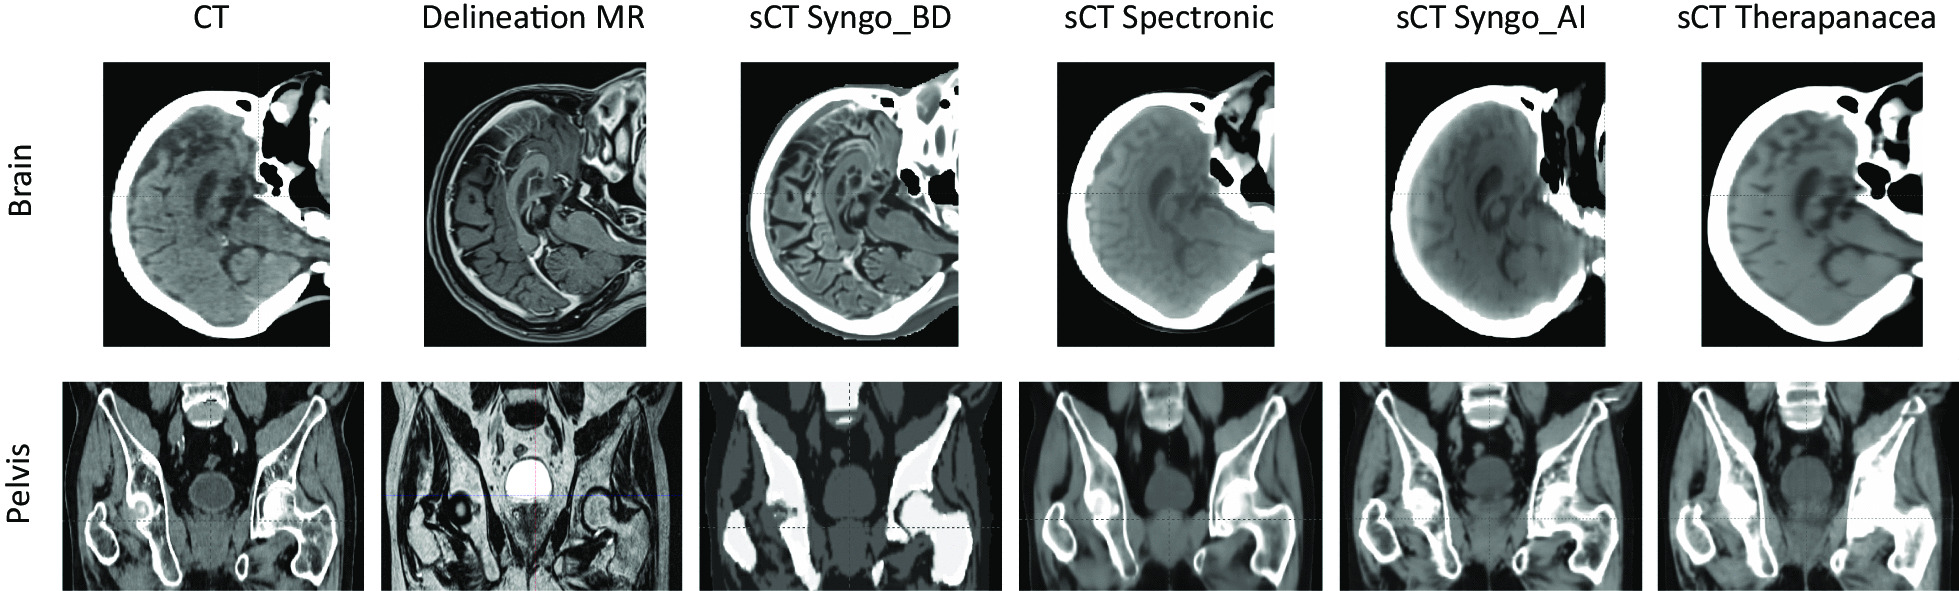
Upper row: example of sagittal images for the CT, MR and sCTs studied of the brain cohort (viewing window for CT and sCT: [ − 20; 100] HU). Lower row: frontal images for the CT, MR and sCTs studied of the pelvis cohort (viewing window for CT and sCT: [ − 125; 225] HU)
